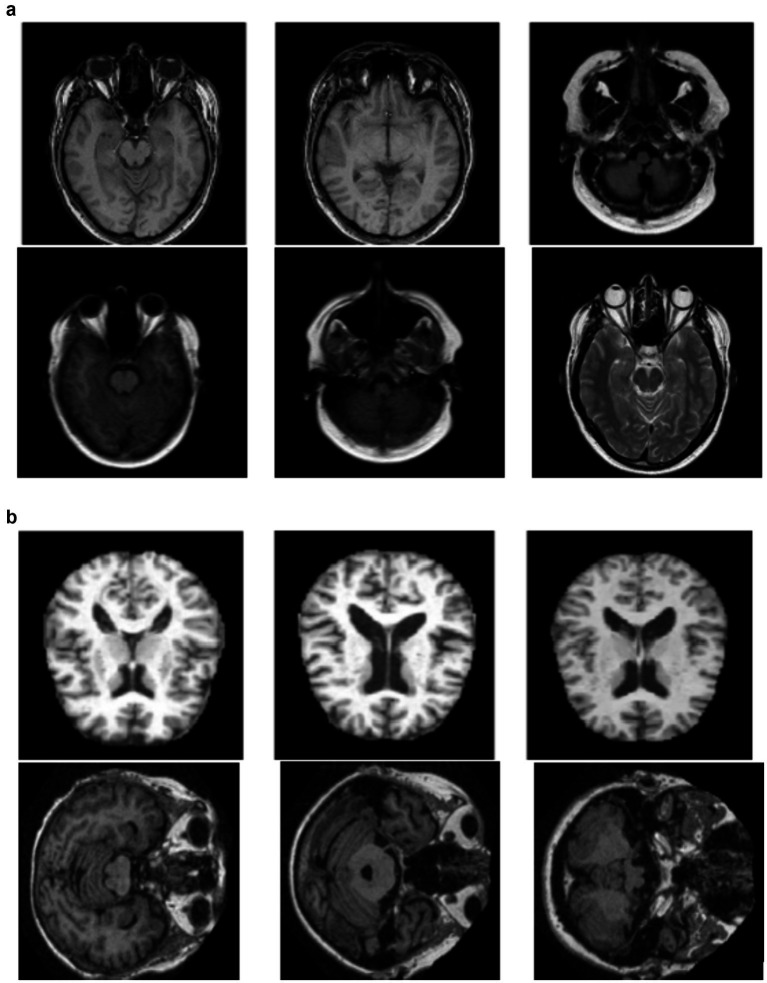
(a) Sample images indicating a non demented Brain MRI scan. (b) Sample images indicating a demented brain-MRI scan.
